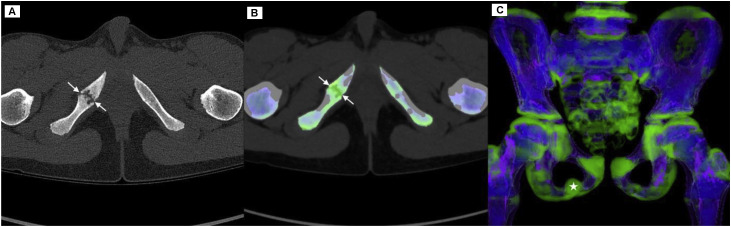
(A) Axial PC-CT (B-C) Axial and coronal PC-CT Q-Peak protocol images with VNC reconstruction showing edema centered on the synchondrosis corresponding to the enhanced lesion in MRI (white arrows in B). Note in the 3D rendering of VNC coronal reconstruction, the obvious asymmetry compared to contralateral branch (asterisk).
